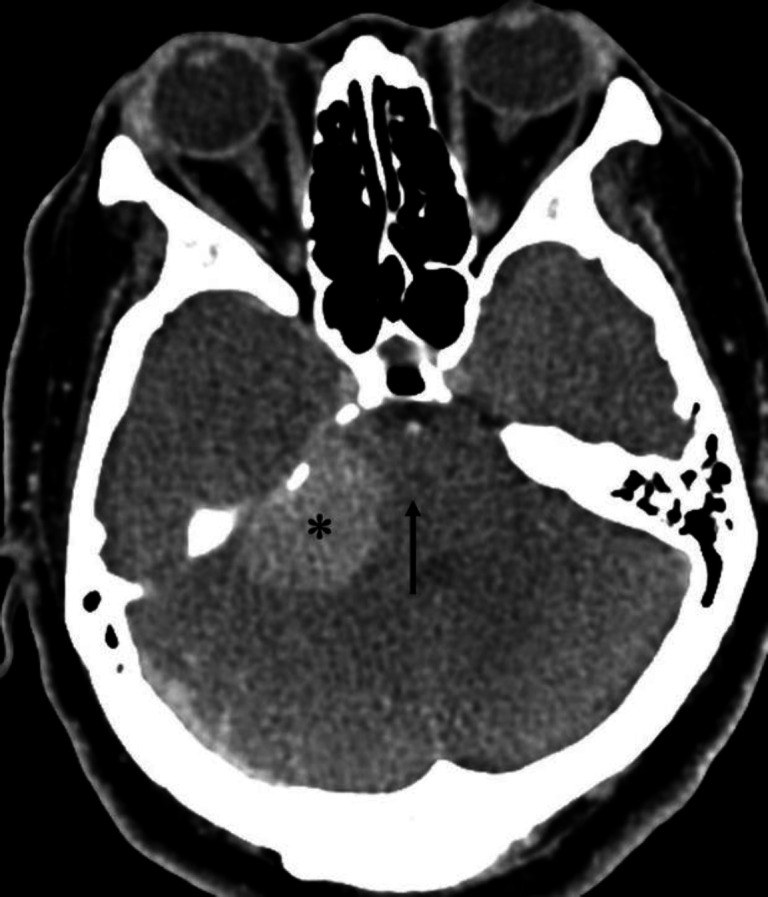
Non-contrast CT brain of a 38 year-old female shows a high density extra-axial mass in the cerebellopontine angle region (asterisk). Note the compression of the pons (black arrow) by the mass. Main differential considerations of an extra-axial mass in this location are meningioma and vestibular schwannoma. It is to be noted that distinction between an intra-axial and extra-axial location of an intracranial mass is often challenging on a single image. Hence, review of all images in different orthogonal places (axial, coronal and sagittal) is necessary for making the distinction
